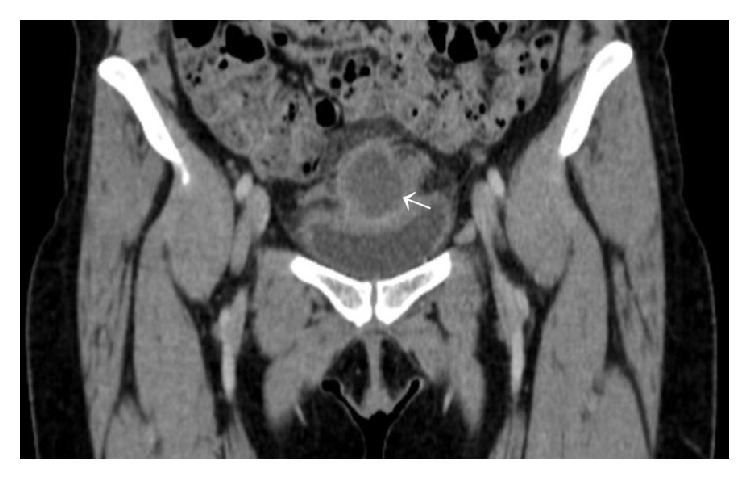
Abdominopelvic computed tomography showing urachal abscess (coronal view).
